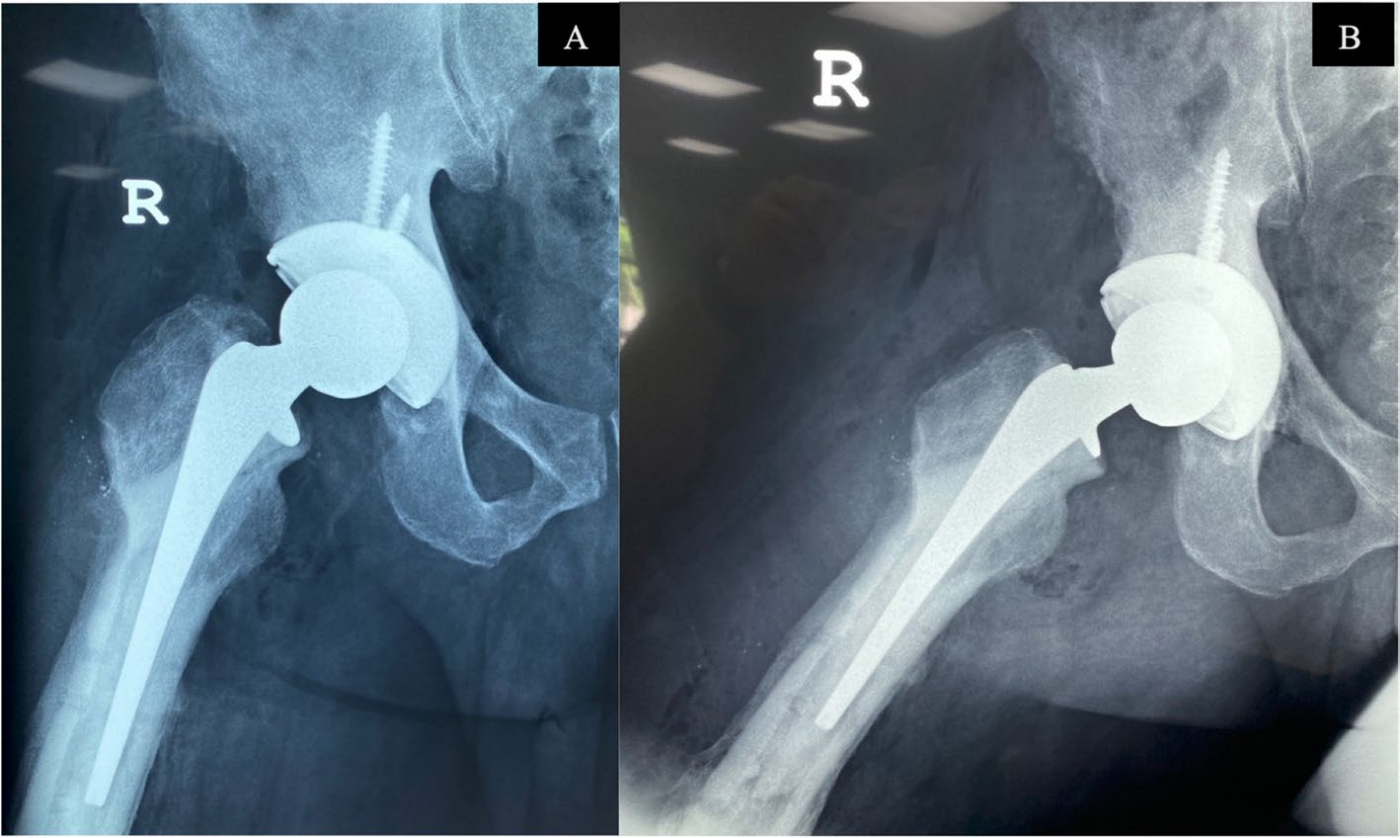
Post-operative anteroposterior (A) and lateral radiographs (B) after removal of all implants and revision to total hip arthroplasty
